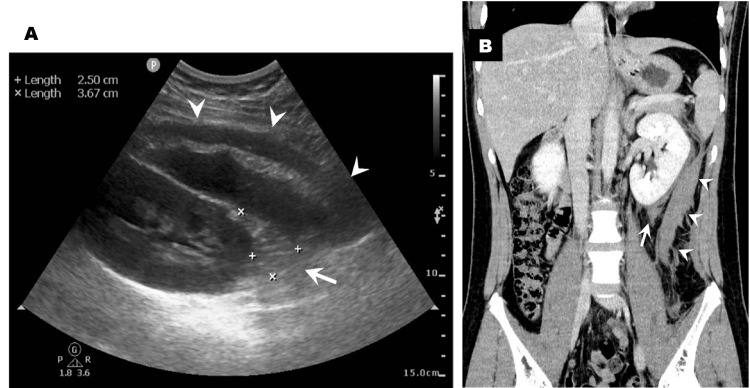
Imaging findings of the patient after renal biopsy. (A) Dorsal view of renal ultrasonography shows retroperitoneal (arrowheads) and perinephric haematomas (arrow). The layer of mixed echogenicity suggests repeated haemorrhage. (B) Abdominal contrast-enhanced computed tomography shows retroperitoneal (arrowheads) and perinephric (arrow) haematomas. The retroperitoneal haematoma (measuring 3 × 7 × 12 cm) extends from the left kidney to the left iliopsoas muscle. Extravasation of contrast media, pseudoaneurysm, or arteriovenous fistula was not detected.
